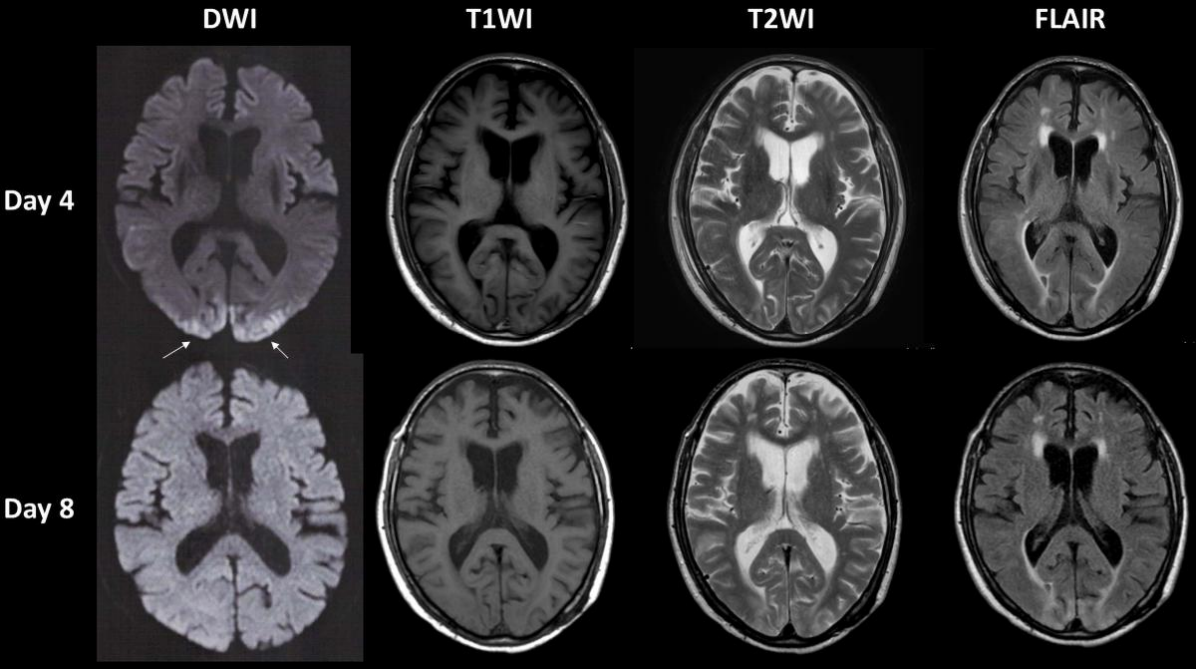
Diffusion-, T1-, and T2-weighted and fluid-attenuated inversion recovery images obtained via magnetic resonance imaging of the head on Days 4 and 8.
